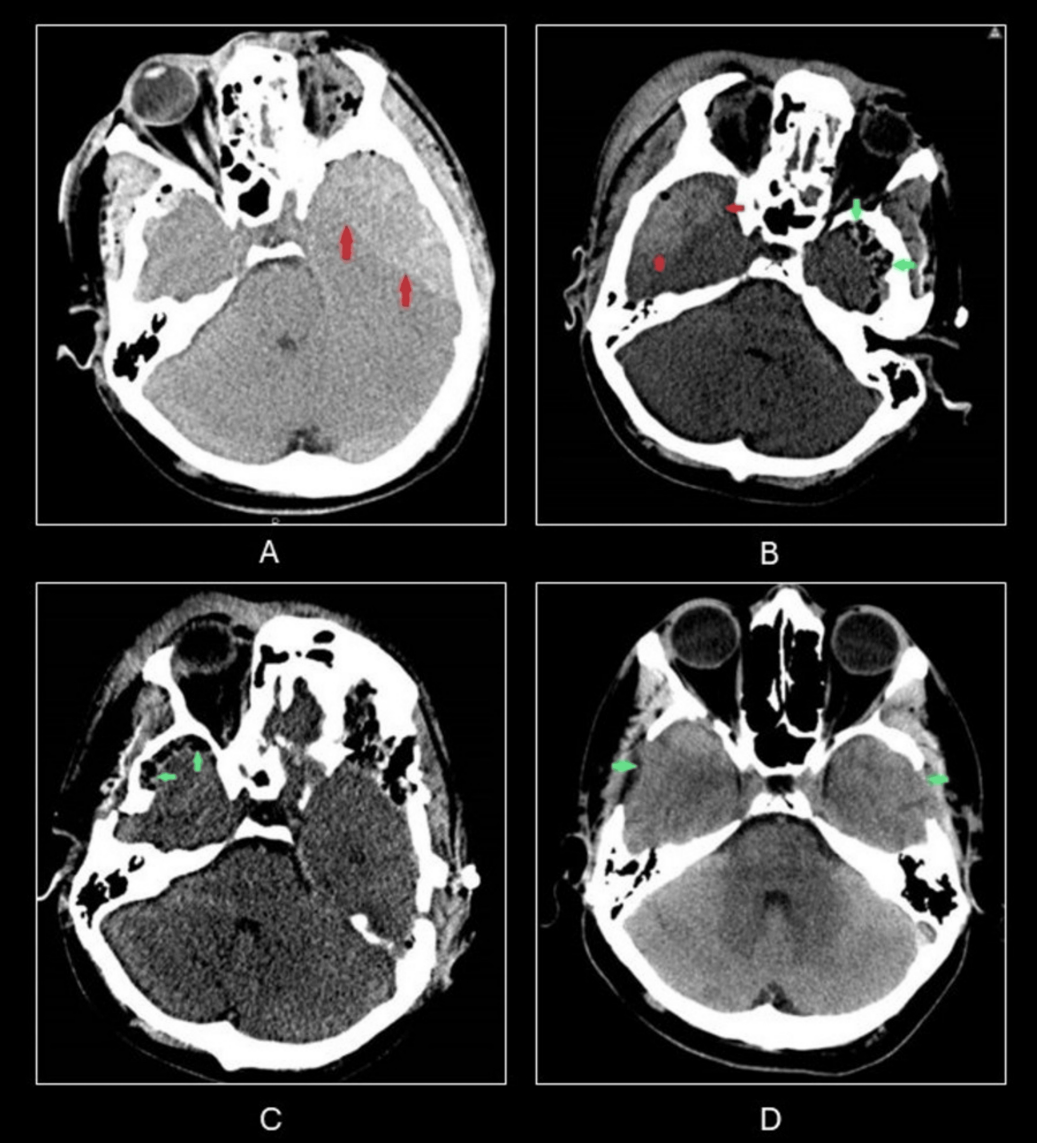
CT scans A:Preoperative CT showing left temporal EDH (red arrows); B:Immediate postoperative CT showing evacuation of left temporal EDH with postoperative changes (green arrows) and new right temporal EDH (red arrows); C:Early postoperative CT showing evacuation of bilateral EDH and postoperative changes (green arrows); D:One-month postoperative CT showing evacuation of bilateral EDH and postoperative changes (green arrows) EDH: epidural hematomas, CT: computed tomography
